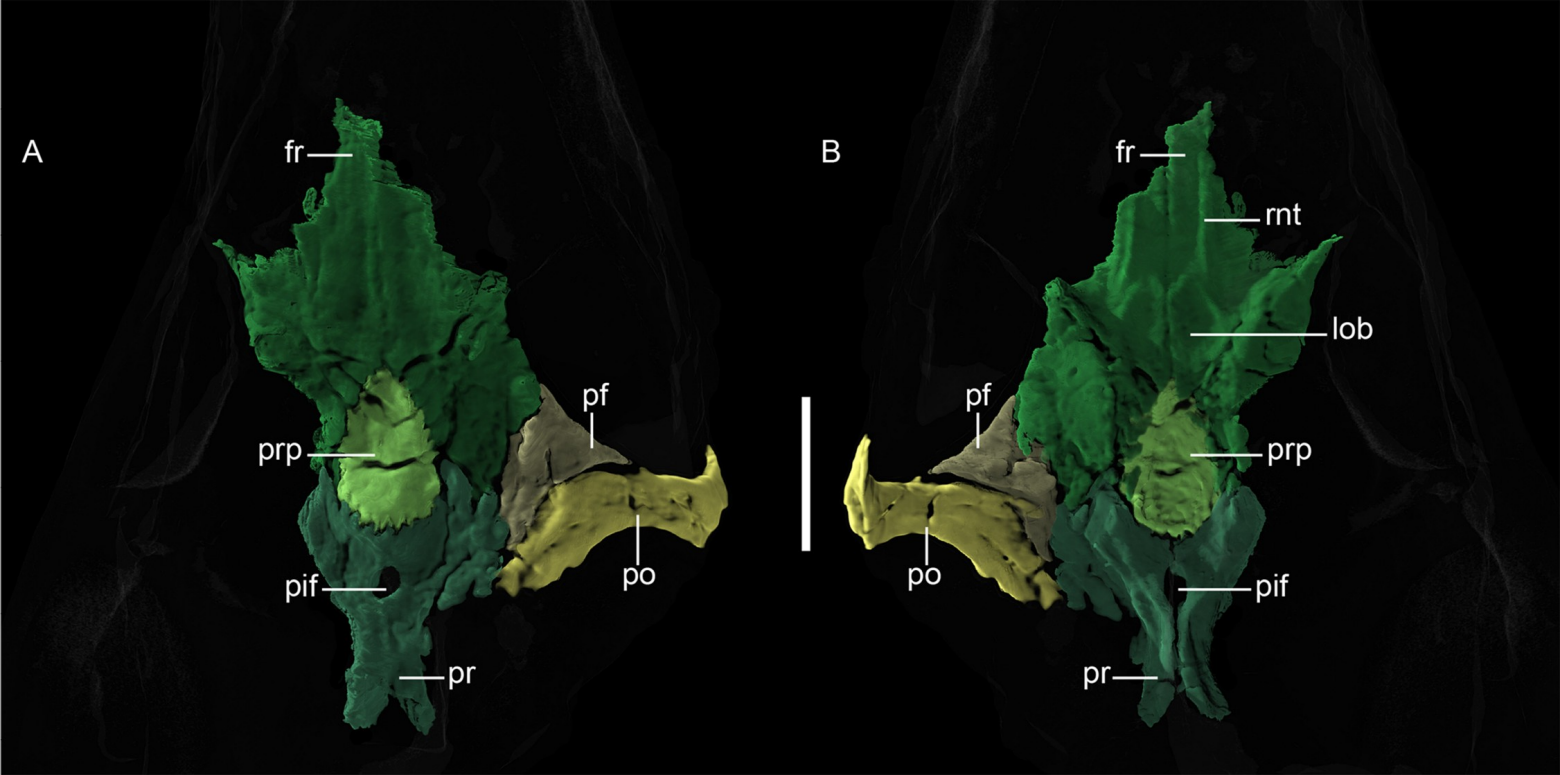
Neutron CT reconstruction of MB.R.999: skull roof from (A) dorsal and (B) ventral. Abbreviations: fr, frontal; lob, (position of) left olfactory bulb; pf; postfrontal; pif, parietal foramen; po, postorbital; pr, parietal; prp, preparietal; rnt: ridge for cartilaginous nasal turbinals. Scale bars = 2 cm.
